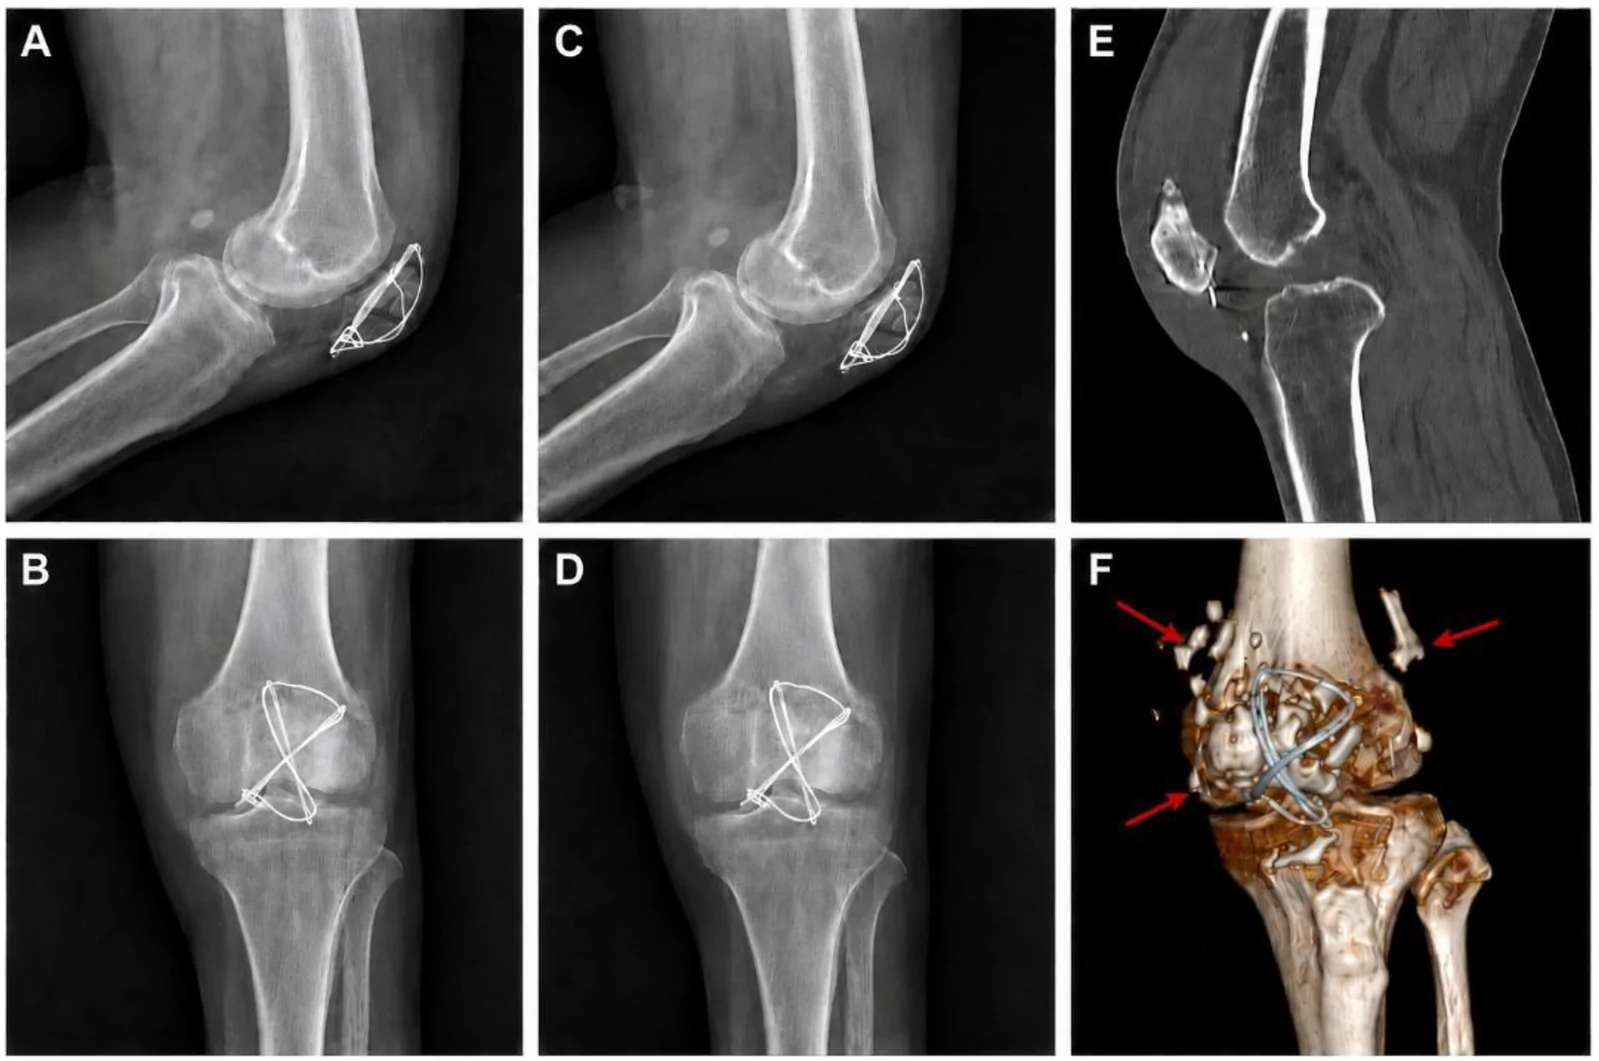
Postoperative radiographic and CT follow-up after revision surgery. (A,B) Lateral and anteroposterior radiographs at 1 month after revision surgery, showing maintained fixation without obvious redisplacement. (C,D) Lateral and anteroposterior radiographs at 2 months after revision surgery, showing no obvious progression of fragment displacement. (E,F) CT images at the final follow-up, demonstrating partial fracture union with residual nonunion and displaced/avulsed bone fragments around the patella.
